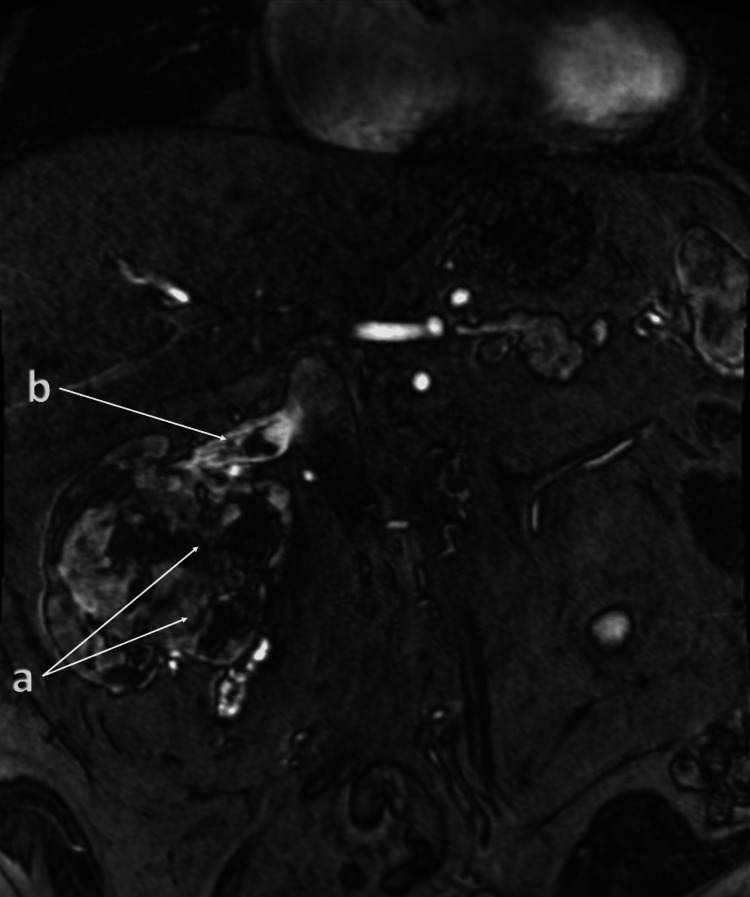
MRI angiogram of the abdomen MRI coronal image showed a large mixed solid and cystic mass (a, arrows) emanating from the right renal pelvis measuring 8.4 × 10.0 × 8.3 cm (AP × TR × CC). A focal filling defect (b, arrow) is seen within the draining right renal vein measuring approximately 2 cm long, demonstrating mild heterogeneous enhancement, and findings are most compatible with tumor thrombus. MRI: magnetic resonance imaging, AP: anteroposterior, TR: transverse, CC: craniocaudal
